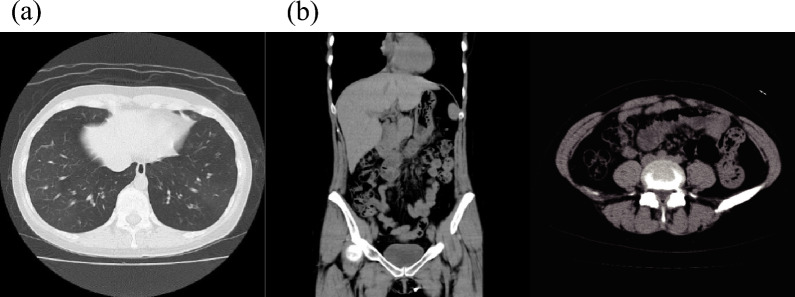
Image findings. a Mild bronchial thickening in chest computed tomography. b No ascites or intestinal wall thickening is noted
